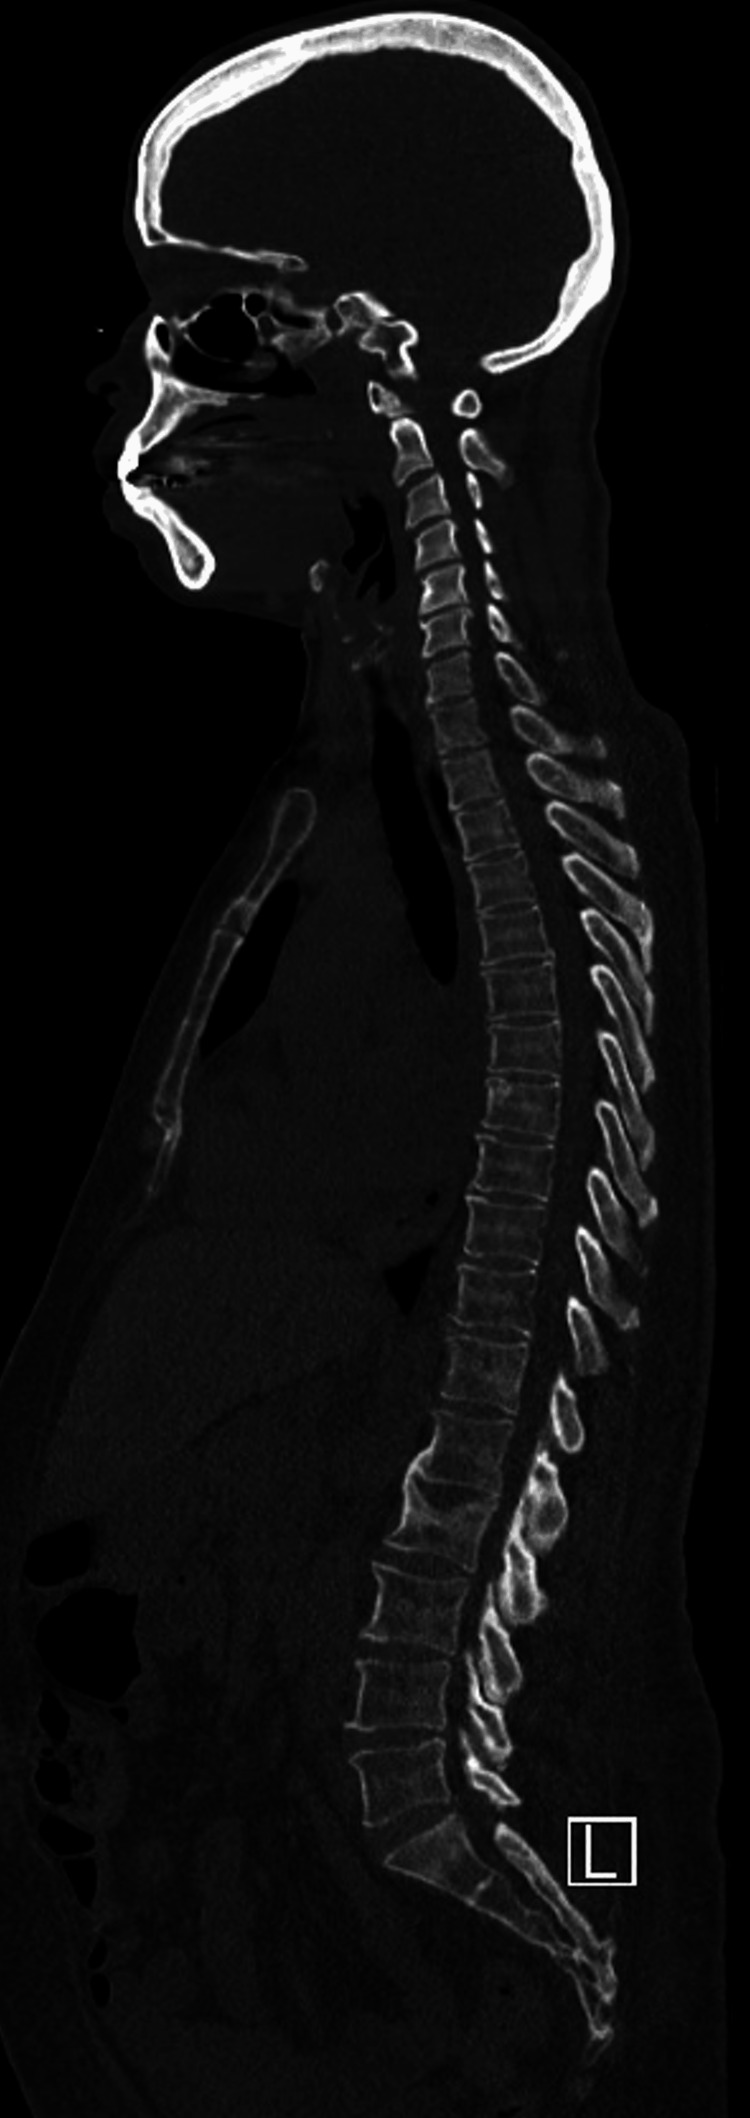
Normal CT Myeloma Survey Sagittal section of the CT myeloma survey, which did not reveal any lytic lesions and was overall unremarkable. Osteosclerotic or mixed sclerotic/lytic lesions are one of the three major criteria for POEMS. In this case, the patient satisfied a major criterion by the presence of elevated plasma VEGF protein. CT: computed tomography; POEMS: polyneuropathy, organomegaly, endocrinopathy, monoclonal gammopathy, and skin changes; VEGF: vascular endothelial growth factor
